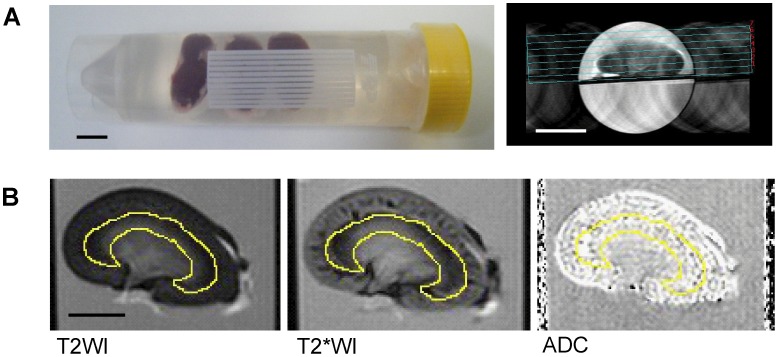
MRI measurement methods for cardiac death (CD) kidney grafts. (A) Left: Harvested kidneys from CD donors soaking in University of Wisconsin (UW) solution. Three left kidneys were attached to plastic plates and soaked in a 4°C UW solution immediately after harvest or perfusion. Scale bar: 1 cm. Right: The sectional positions of longitudinal kidney MRI images. To get the mid longitudinal plane image of a kidney graft, series of MRI images were taken in parallel with the plastic plate. Scale bar: 1 cm. (B) MRI images of selected regions referenced by T_2_ weighted images. T_2_* signal intensity (SI) and apparent diffusion coefficient (ADC) were measured in the same regions referenced T2weighted images by Image J software. Scale bar: 0.5 cm.
